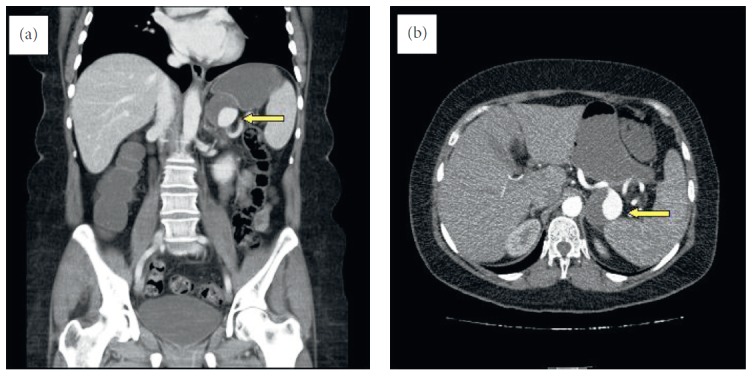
Multislice computed tomographic image of the splenic artery aneurysm.
